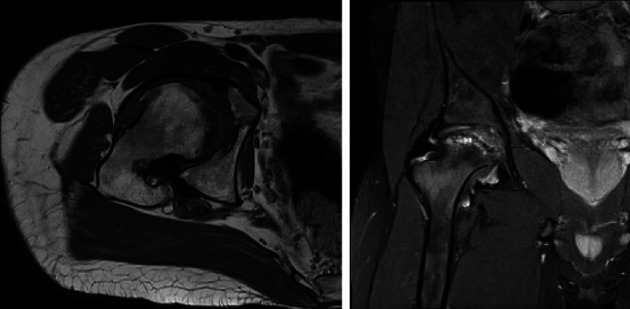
MRI of the right hip at the age of 22 years. The left image depicts the femoral head on the axial plane in a fat-saturated sequence. Edema can be observed in the femoral head. The right image shows the hip in coronal plane in a T1 sequence after application of contrast medium (gadolinium). The femur head has been deformed into a sickle-like shape.
